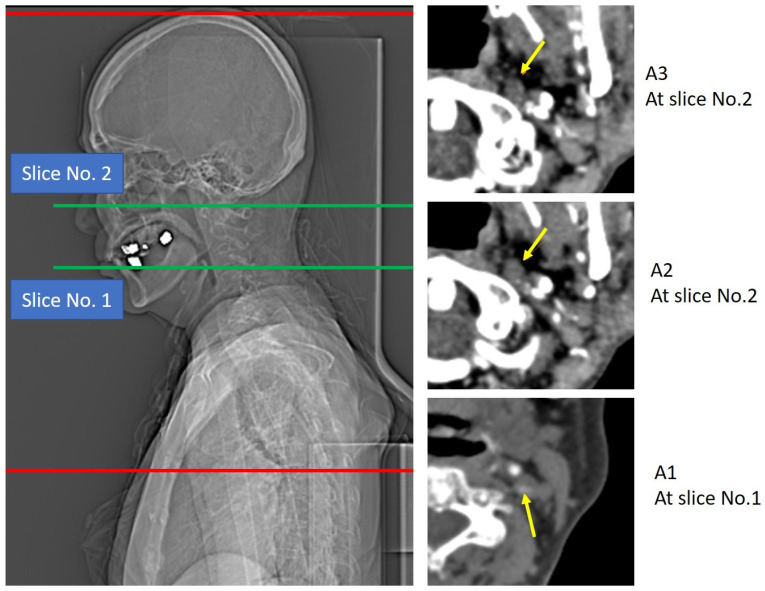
This case demonstrates the highest level of contrast enhancement at the proximal cervical segment of the left internal carotid artery during the first arterial phase (A1). In the second (A2) and third (A3) arterial phases, however, the limited z-axis coverage of the standard multiphase CTA, which does not extend to the carotid bifurcation, poses challenges for accurate image interpretation. The yellow arrows indicate the corresponding location of the ICA in A1, A2, and A3, respectively. Notably, Slice No. 2 corresponds to the skull base landmark routinely used to define the lowest z-axis level covered in the second and third arterial phases of the standard multiphase CTA protocol. This highlights the importance of adequate coverage to avoid misinterpretation of arterial occlusion or pseudo-occlusion.
